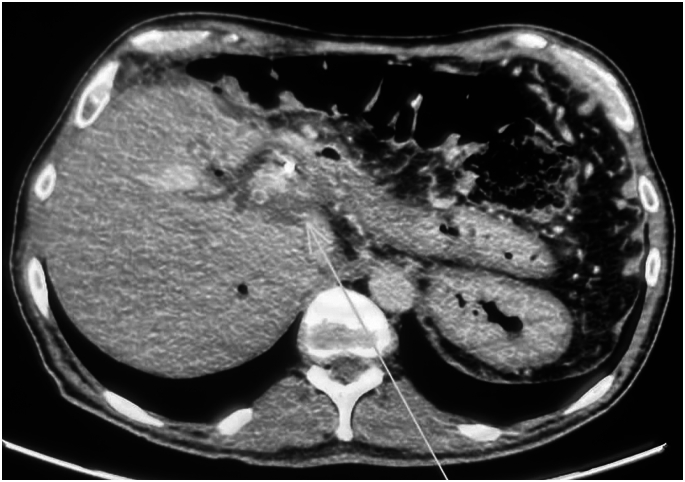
Computed tomography after completion of endoscopic vacuum therapy with no signs of hepatic ischemia.
